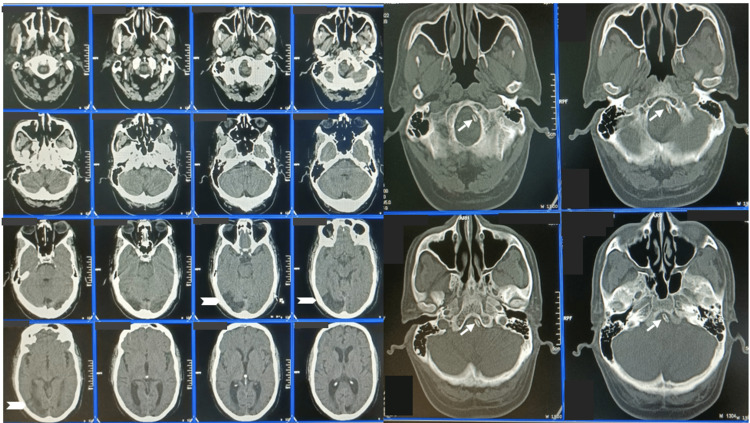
Post-procedural CT changes comparable with the pre-procedural MRI (notched arrow). The stent is seen in the bone window of post-procedure CT (arrow).
